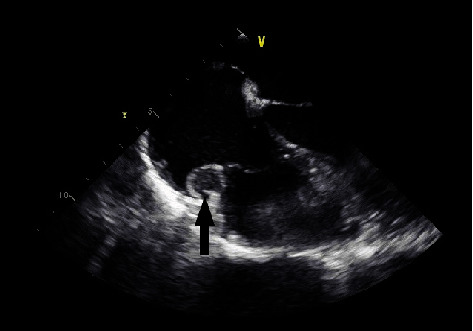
TEE horizontal view: right atrial mass (black arrow) in the area adjacent to the tricuspid valve. No visual obstruction of flow was identified on Doppler.
